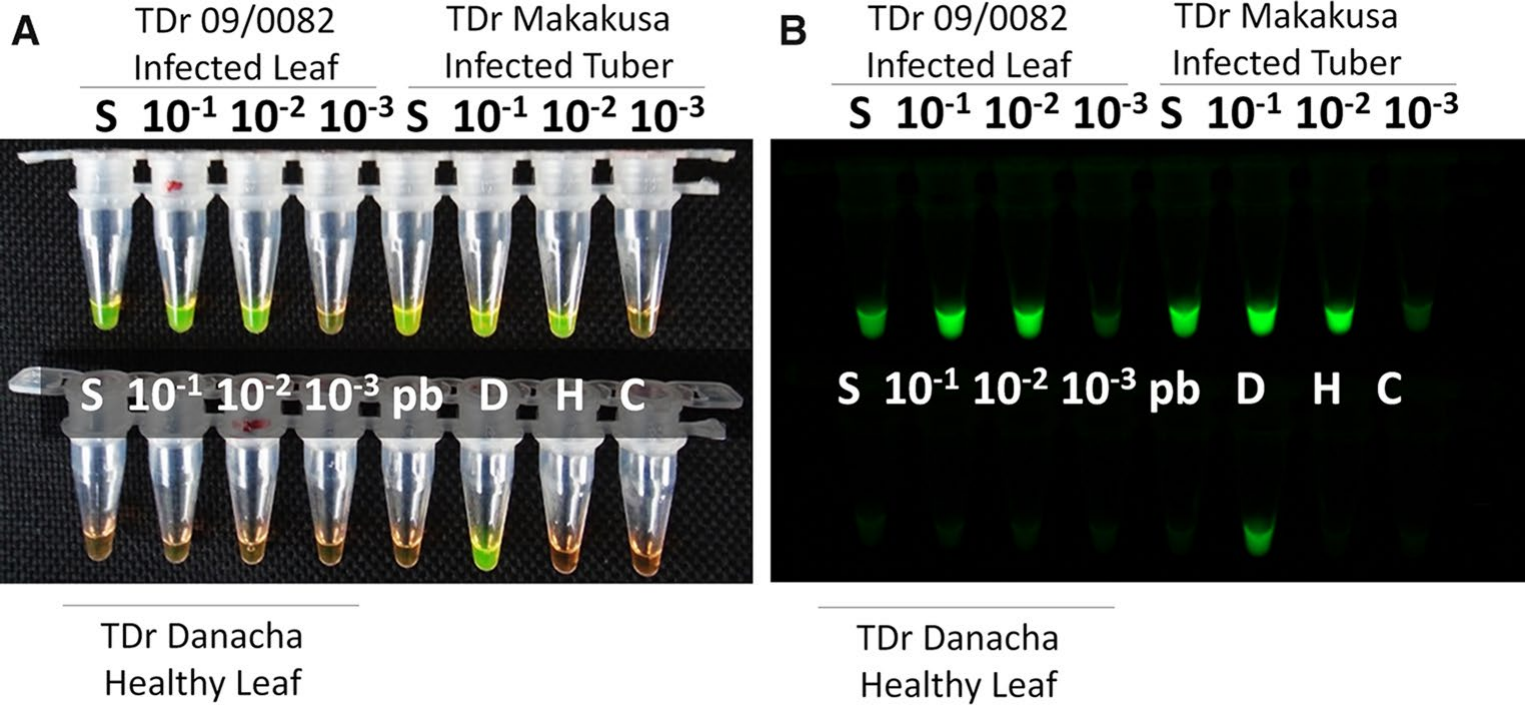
YMV detection in leaf and tuber sap samples by CT-RT-LAMP. Sap was extracted from 100 mg of tissue in alkaline PEG buffer (1:20 dilution), and 2 µl was used as template for virus detection. The genotype number of the yam, template dilution, and infection status are indicated. Pb, alkaline PEG buffer control; D, YMV positive control; H, healthy yam control; B, no-template control; S, undiluted stock
